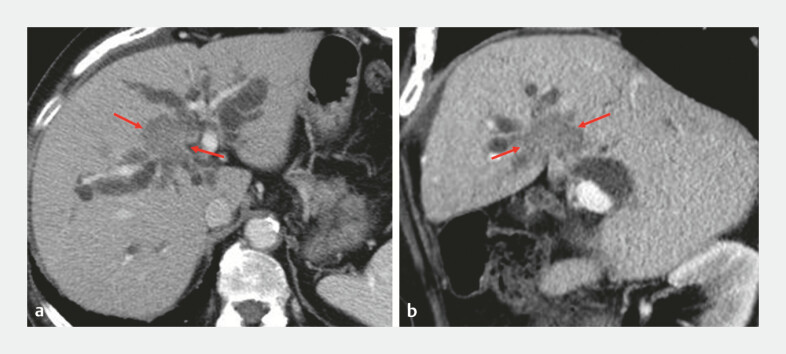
Computed tomography revealing the biliary stricture in the hilar bile duct (a red arrow).
